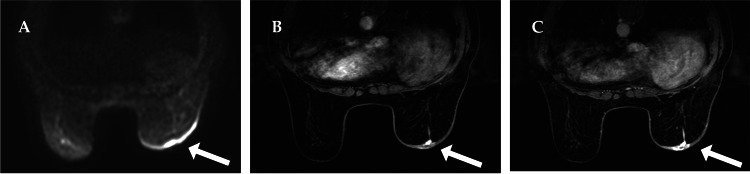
Contrast-enhanced magnetic resonance imaging (MRI) of the breast findings A: Right breast skin thickening with high signal along the thickened area (STIR/DWI). B: In the dynamic study, there was a strong enhancement effect in the subcutaneous area in the form of dots and an enhancement effect predominantly at the limbus on the skin surface (early phase). C: Prolonged enhancing effect (late phase).
